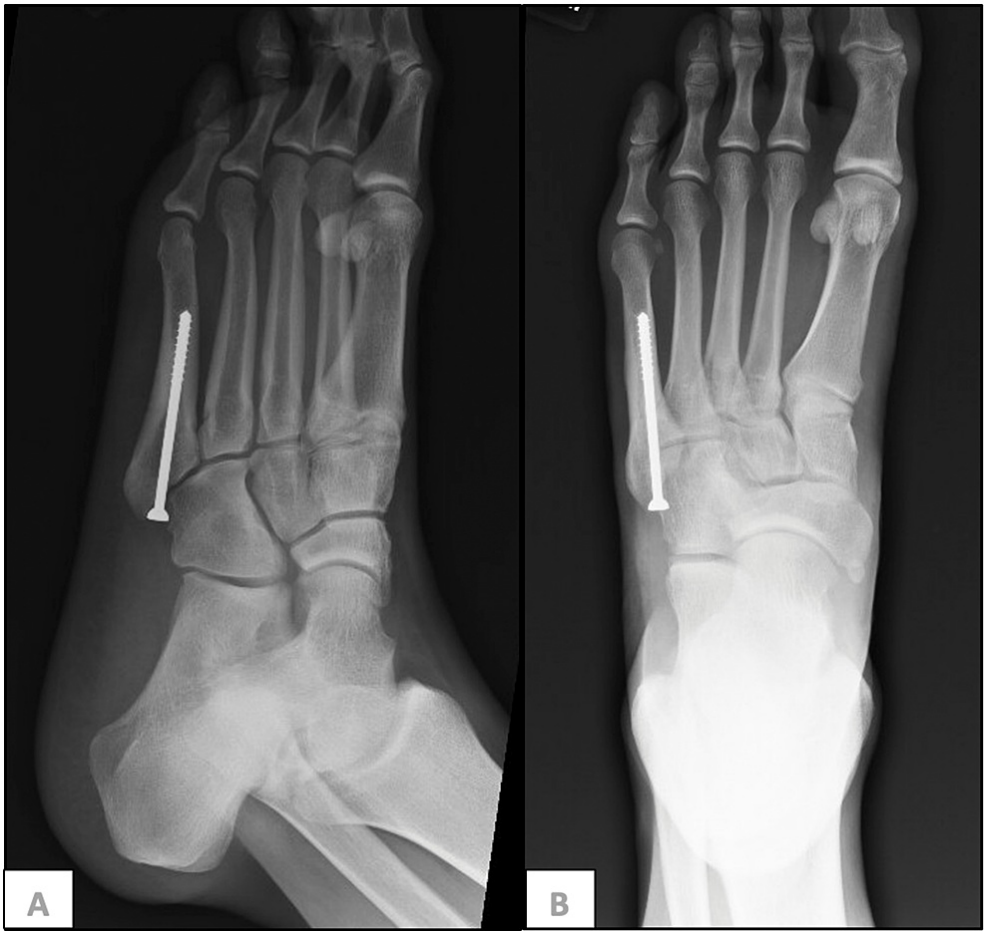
Post-surgical X-rays X-rays of stable screw fixation and post-surgical changes of the fifth metatarsal, without radiographic evidence of recurrent tumor or hardware complications. (A) Anterolateral view; (B) anterior view.
